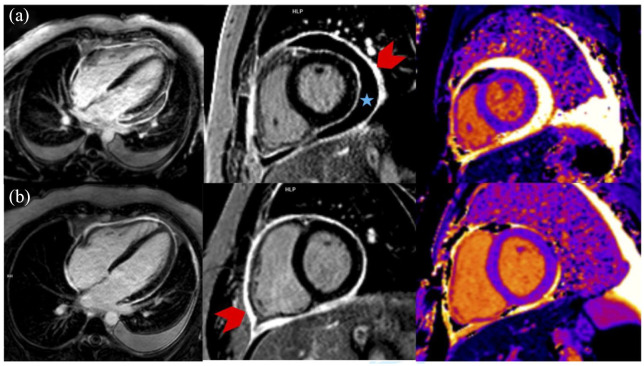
Cardiac MRI: late gadolinium enhancement (LGE) in four-chamber view and short-axis view (SAX). T1 relaxation times as color-coded maps (T1maps) in SAX. Pericardial effusion (blue asterisk) and pericardial LGE (red arrow) indicating pericarditis (a; 2018/08). Increased pericardial thickening and LGE with decrease in pericardial effusion (b; 2019/01). Absent myocardial LGE and normal relaxation times in T1maps, so there are no more signs of previous myocardial involvement. Note the left-sided pleural effusion.
